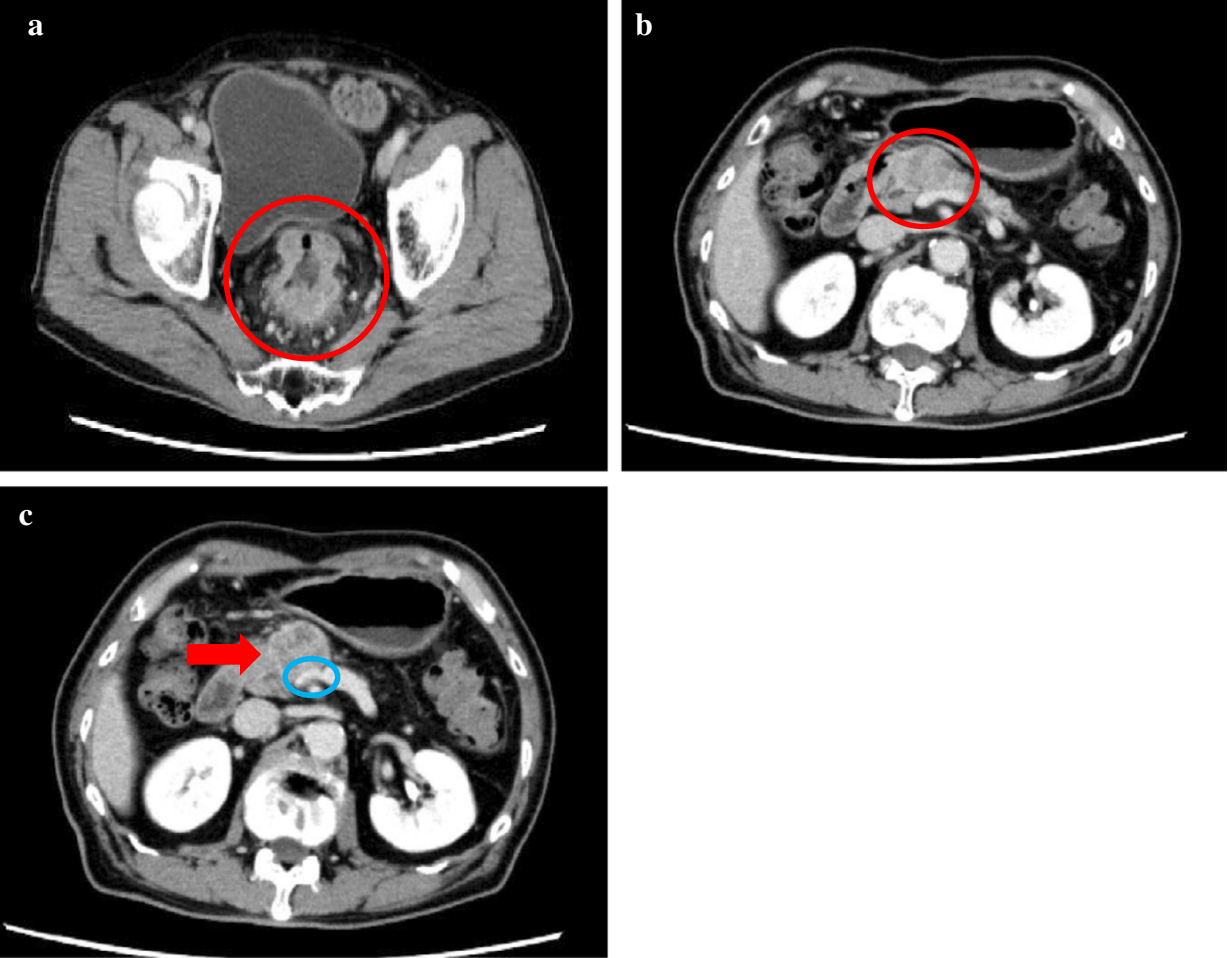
Computed tomography findings at presentation. a Preoperative contrast-enhanced computed tomography showed a clearly enhanced rectal lesion in Ra (red circle) with no lymphadenopathy or distant metastasis. b Contrast-enhanced computed tomography also revealed a poorly enhanced tumor 3 cm in size in the pancreatic head (red circle). c The pancreatic tumor (red arrow) widely abutted the superior mesenteric vein (blue circle)
